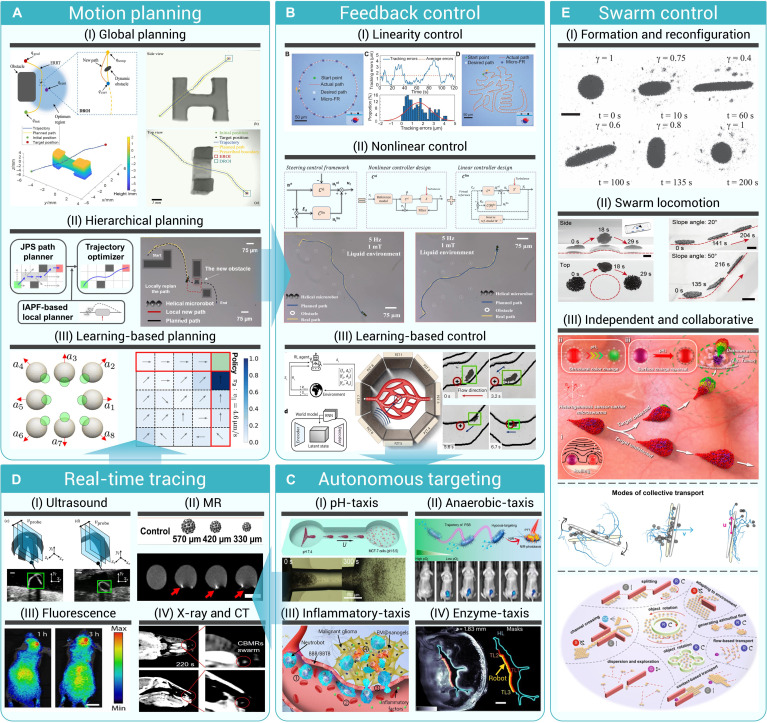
Motion navigation control. (A) Motion planning algorithms. (I) The global path planned by the enhanced rapidly exploring random tree algorithm. Scale bar: 1 mm. Reproduced or adapted with permission from Ref. [92], Copyright 2022 IEEE. (II) Global planning and local emergency replanning. Scale bar: 75 μm. Reproduced or adapted with permission from Ref. [44], Copyright 2024 IEEE. (III) Reinforcement learning-based motion planning. Reproduced or adapted with permission from Ref. [101], Copyright 2021 AAAS. (B) Motion feedback control. (I) Linear proportional-integral-derivative (PID) control. Scale bar: 50 μm. Reproduced or adapted with permission from Ref. [102], (CC BY-NC 4.0). (II) Compound nonlinear control. Scale bar: 75 μm. Reproduced or adapted with permission from Ref. [105], Copyright 2024 IEEE. (III) Reinforcement learning control. Scale bar: 200 μm. Reproduced or adapted with permission from Ref. [111], (CC BY 4.0). (C) Autonomous targeting motion. (I) Microrobots migrate in response to local pH gradients. Scale bar: 500 μm. Reproduced or adapted with permission from Ref. [114], (CC BY 4.0). (II) Anaerobic bacteria selectively target hypoxic regions. Reproduced or adapted with permission from Ref. [116], Copyright 2020 ACS. (III) Microrobots move along the concentration gradient of inflammatory cytokines. Reproduced or adapted with permission from Ref. [71], Copyright 2021 AAAS. (IV) Nanorobots aggregate in response to urease concentration gradients. Scale bar: 500 μm. Reproduced or adapted with permission from Ref. [118], (CC BY 4.0). (D) Visual feedback. (I) Ultrasound imaging. Scale bar: 1 mm. Reproduced or adapted with permission from Ref. [77], (CC BY 4.0). (II) Magnetic resonance (MR) imaging. Scale bar: 5 mm. Reproduced or adapted with permission from Ref. [86], (CC BY-NC 4.0). (III) Fluorescence imaging. Scale bar: 10 mm. Reproduced or adapted with permission from Ref. [71], Copyright 2021 AAAS. (IV) Computed tomography (CT) imaging. Scale bar: 2 cm. Reproduced or adapted with permission from Ref. [89], Copyright 2025 AAAS. (E) Swarm control. (I) Various swarm formations. Scale bar: 500 μm. Reproduced or adapted with permission from Ref. [136], Copyright 2022 IEEE. (II) Directional movement of a swarm. Scale bar: 5 mm. Reproduced or adapted with permission from Ref. [138], (CC BY-NC 4.0). (III) Independent and collaborative tasks performed by multiple swarms. Reproduced or adapted with permission from Ref. [140], Copyright 2024 ACS; [133], Copyright 2024 AAAS; [141], (CC BY-NC 4.0). BBB, blood–brain barrier.
